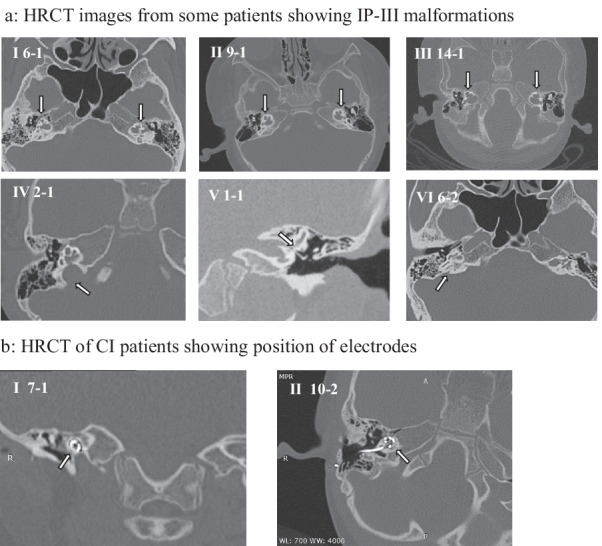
HRCT images of temporal bone and MRI of inner ear in some IP-III patients. a HRCT images from some patients showing IP-III malformations. I-III: “Cloudy like” characteristics of the cochlea: absence of cochlear modiolus and bony spiral lamina, and direct intercommunication between cochlea and IAC; IV: High jugular bulb; VIII-IX: Thickened stapes footplate; V: Visible vestibular aqueduct. b HRCT of CI patients showing position of electrodes. I: Coronal scan of patient 7–1; II: Axial scan of patient 10–2
